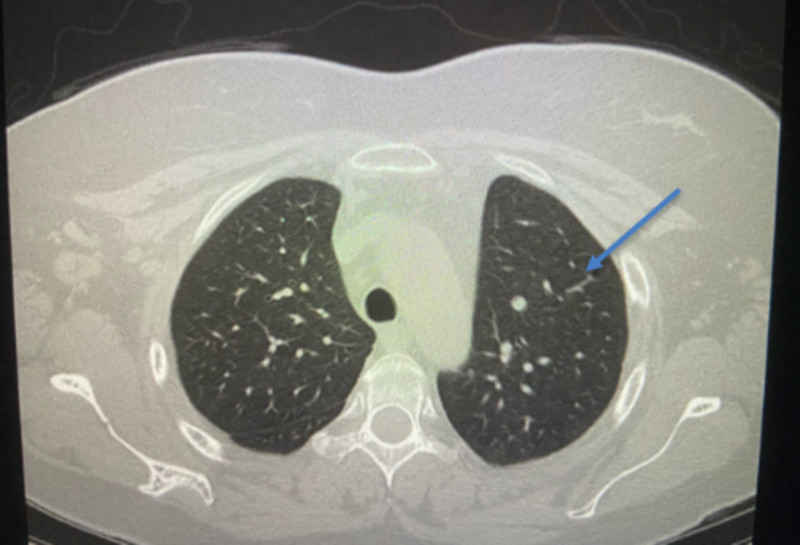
CT scan of chest without contrast revealing normal pulmonary parenchyma.
